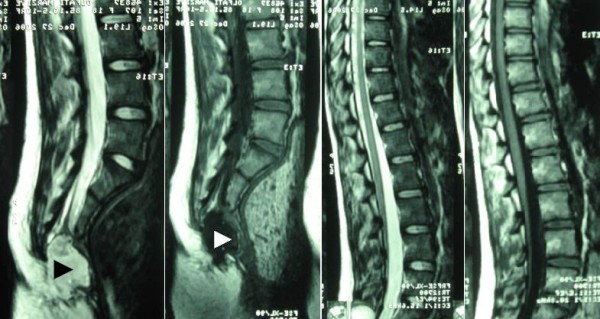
Sagittal magnetic resonance images demonstrating anterior sacral meningocele originating from the caudal thecal sac (arrowheads), associated with thick tethered filum terminale.
